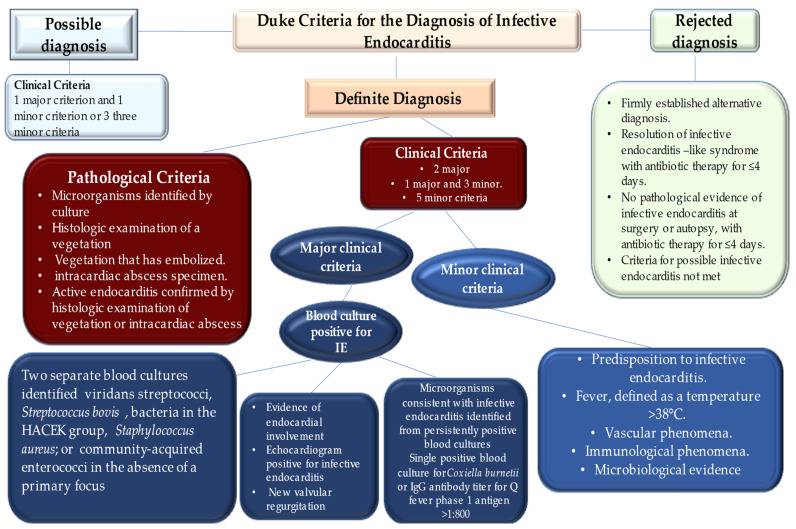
Depiction of Duke criteria for the diagnosis of infective endocarditis. HACEK indicates haemophilus species, *Aggregatibacter* (formerly *Actinobacillus*) *actinomycetemcomitans*, *Cardiobacterium hominis*, *Eikenella corrodens*, and *Kingella kingae*. For patients who have prosthetic valves and possible infective endocarditis according to clinical criteria, transoesophageal echocardiography is recommended. For patients with native valve endocarditis, transthoracic echocardiography is recommended.
